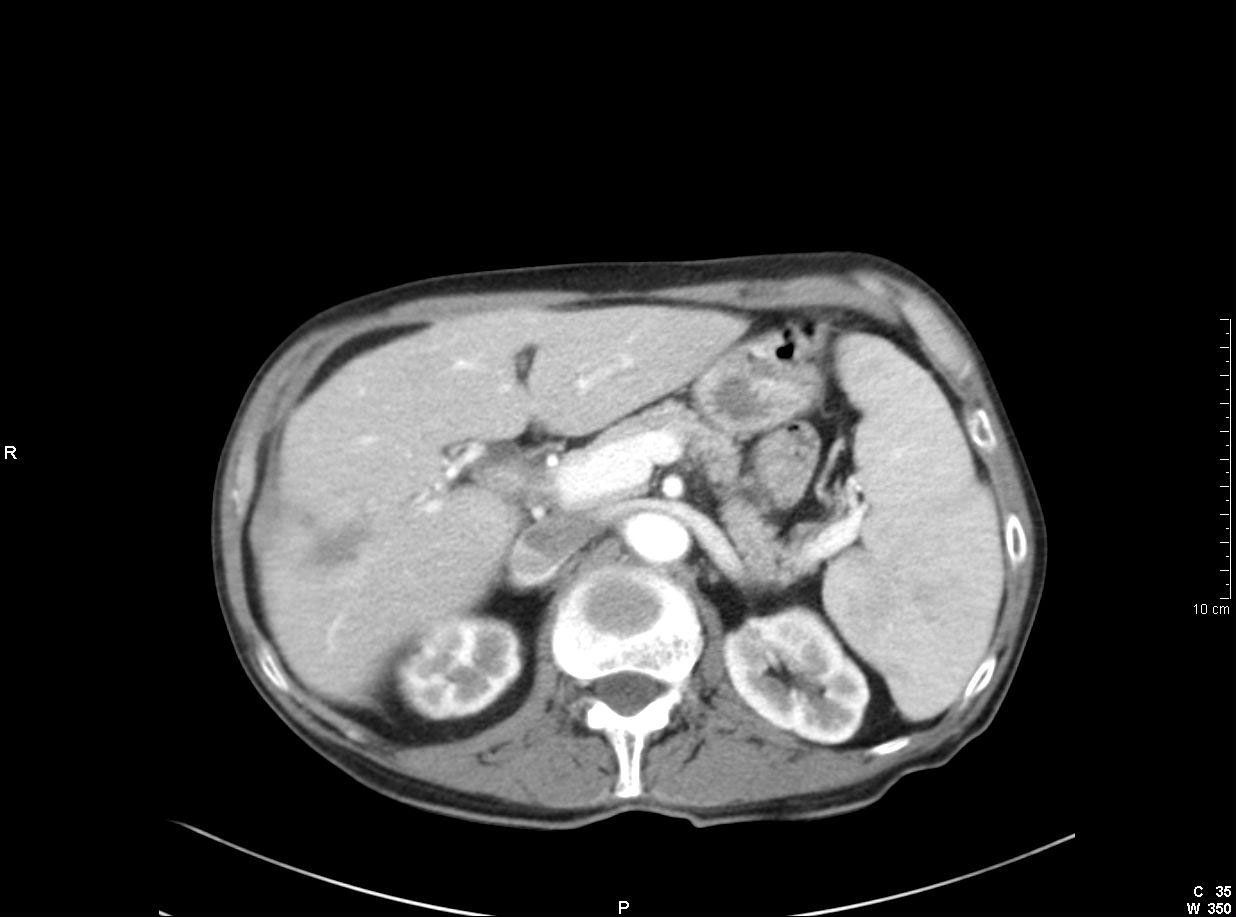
CT scan showing a marked reduction in size of the hepatic lesions. A small organised subcapsular haematoma is noted as result of previous biopsy.
